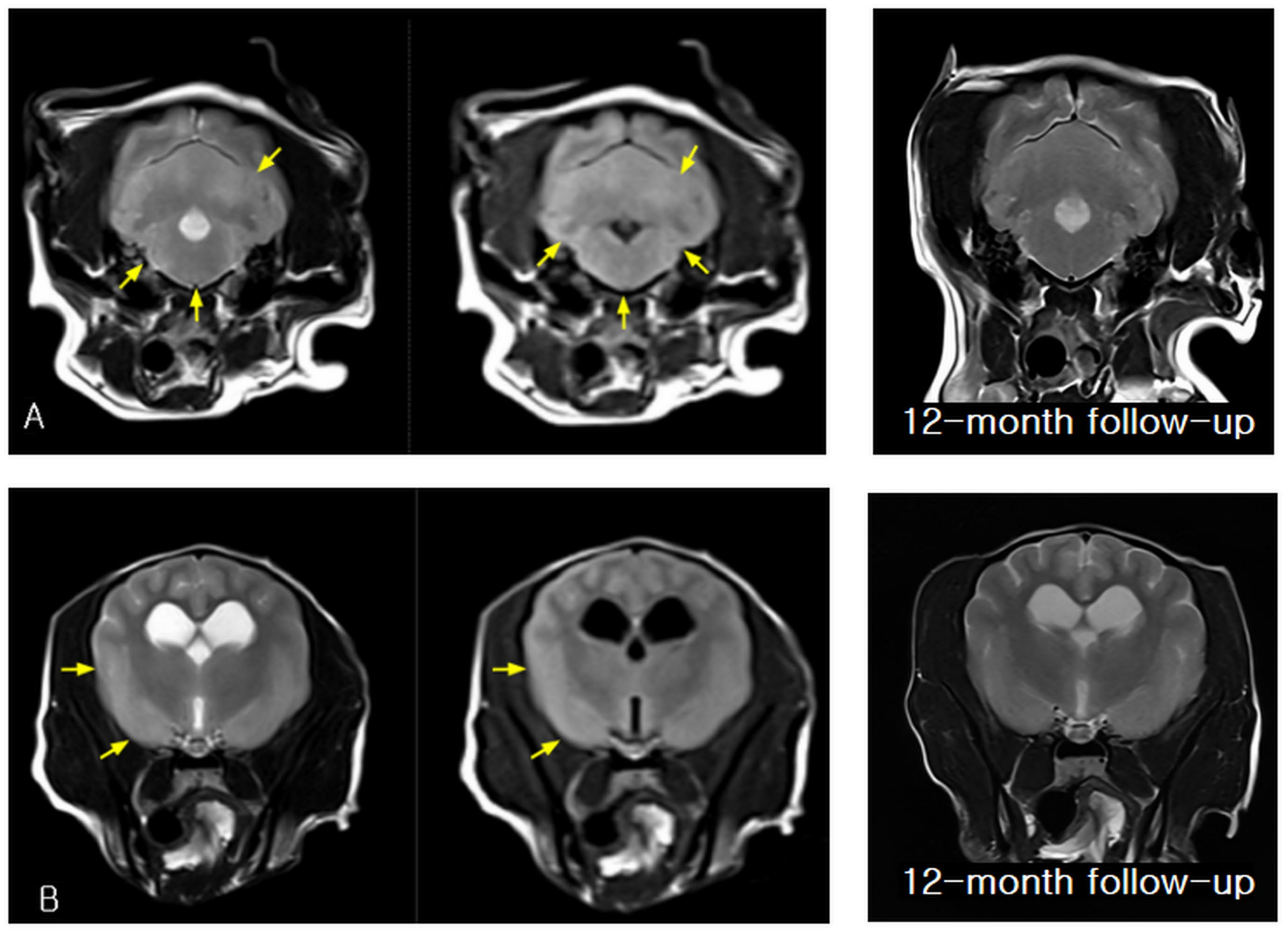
Baseline and 12-month follow-up magnetic resonance imaging (MRI) findings obtained after initiation of steroid-free immunosuppressive therapy, demonstrating resolution of inflammatory lesions involving the brainstem and forebrain. (A) Baseline transverse T2-weighted and fluid-attenuated inversion recovery (FLAIR) images showing bilateral, poorly marginated hyperintensity involving the pons and medulla, consistent with inflammatory parenchymal involvement of the caudal brainstem. At the 12-month follow-up, a transverse T2-weighted image obtained at the same anatomical level demonstrates normalization of parenchymal signal intensity within the pons and medulla, with no evidence of residual abnormal signal. (B) Baseline transverse T2-weighted and FLAIR images of the forebrain demonstrating mild right frontal cortical and subcortical hyperintensity, indicating rostral extension of the inflammatory process. At the 12-month follow-up, a transverse T2-weighted image of the forebrain shows normal cortical and subcortical signal intensity, with resolution of the previously observed right frontal abnormalities and no abnormal signal intensity or structural distortion.
